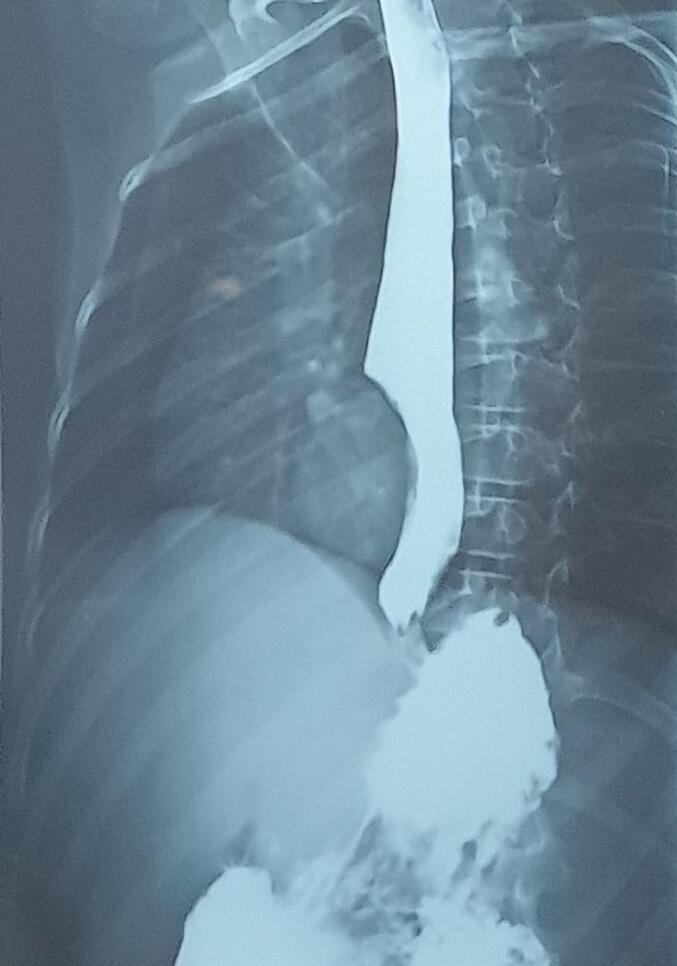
Chest X-ray opaque oval shape in favor of a lower middle mediastinal mass.
